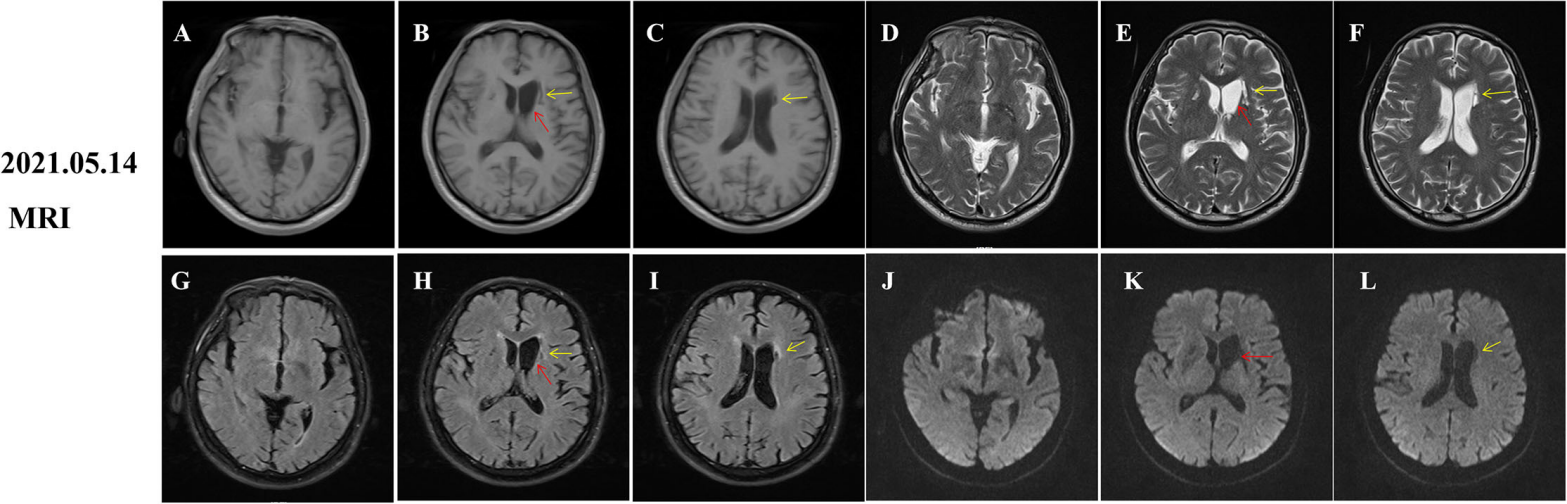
Follow-up magnetic resonance imaging (MRI) of brain 18 months after onset. Diabetic striatopathy (DS)-associated striatal hyperintensity on T1 and T2 and fluid-attenuation inversion recovery (FLAIR) images were disappeared (A,D,G,J), but atrophy of the left caudate nucleus with resultant dilatation of frontal horn of left lateral ventricle was noted [(B,E,H,K) red arrows]. Remote cerebral infarctions were observed on T1, T2, FLAIR and diffusion weighted imaging (DWI) images [(B,C,E,F,H,I,K,L), yellow arrows].
